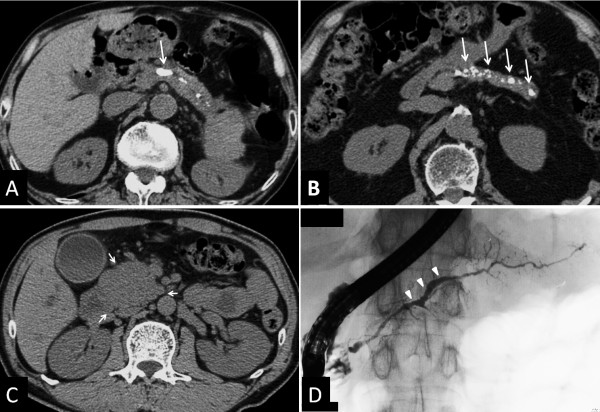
Images of AIP exhibiting definite chronic pancreatitis, and findings of AIP demonstrating independent risk factors for progression to chronic pancreatitis. (A) CT image of stones in pancreatic ducts (arrow). (B) CT image of multiple or numerous calcifications distributed throughout the entire pancreas (arrows). CT and ERCP findings of AIP demonstrating independent risk factors for progression to confirmed chronic pancreatitis at diagnosis. (C) CT finding of pancreatic head swelling at AIP diagnosis (arrows). (D) ERP finding of MPD non-narrowing in the pancreatic body at AIP diagnosis (arrowheads). (From Ref. [54]).
